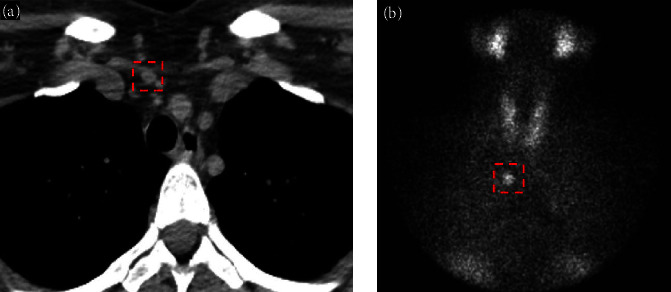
Transversal view of the CT scan (a) highlighting the PTH secreting adenoma at the right upper chest level, along with (b) parathyroid scintigraphy showed a focus of increased 99mTc-sestamibi uptake corresponding to an ectopic parathyroid functioning adenoma.
